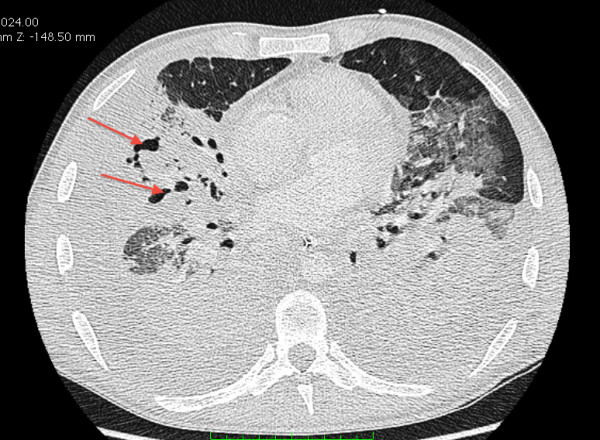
Lung CT scan on day 12 of hospitalization showing abscess formations in the right middle lobe (arrows).
